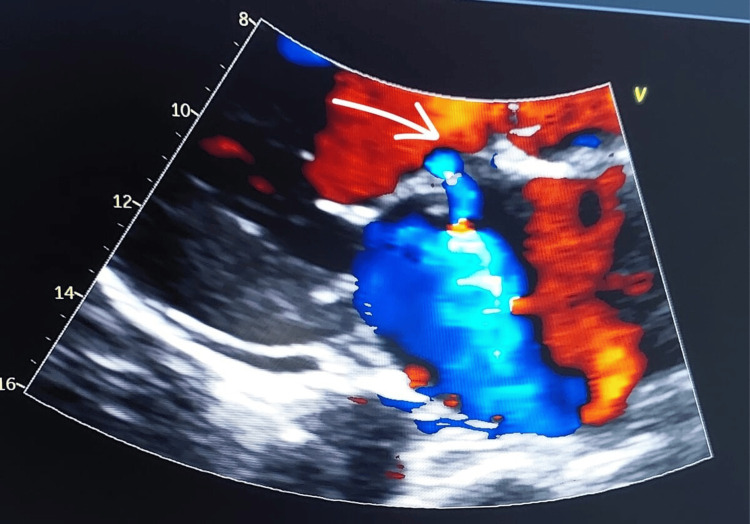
Transthoracic echocardiography (TTE) scan, showing small perforation of anterior mitral valve leaflet (arrow), a complication of infective endocarditis
